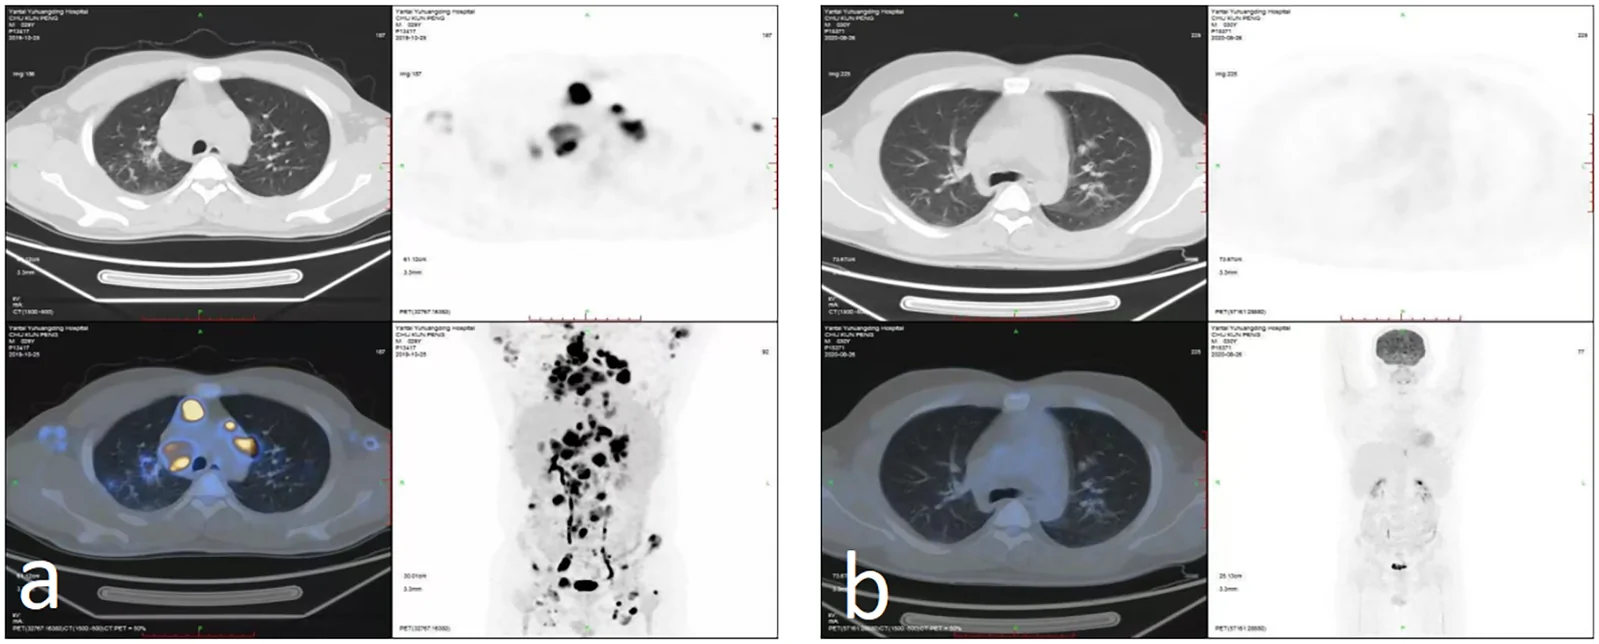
Imaging examinations before and after treatment. (A) Whole-body PET-CT shows uneven thickening of the bilateral and posterior walls of the nasopharynx; multiple lymph nodes throughout the body, including bilateral preauricular, right retroauricular, and bilateral cervical regions, exhibit intense FDG uptake; (B) PET-CT reveals no abnormal FDG metabolic lesions.
